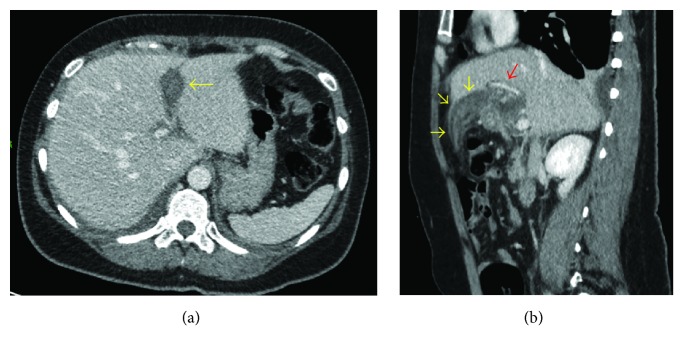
Abdominal axial (a) and sagittal (b) CT scan showing thrombosed falciform ligament (yellow arrows) with patent left portal vein (red arrow).
